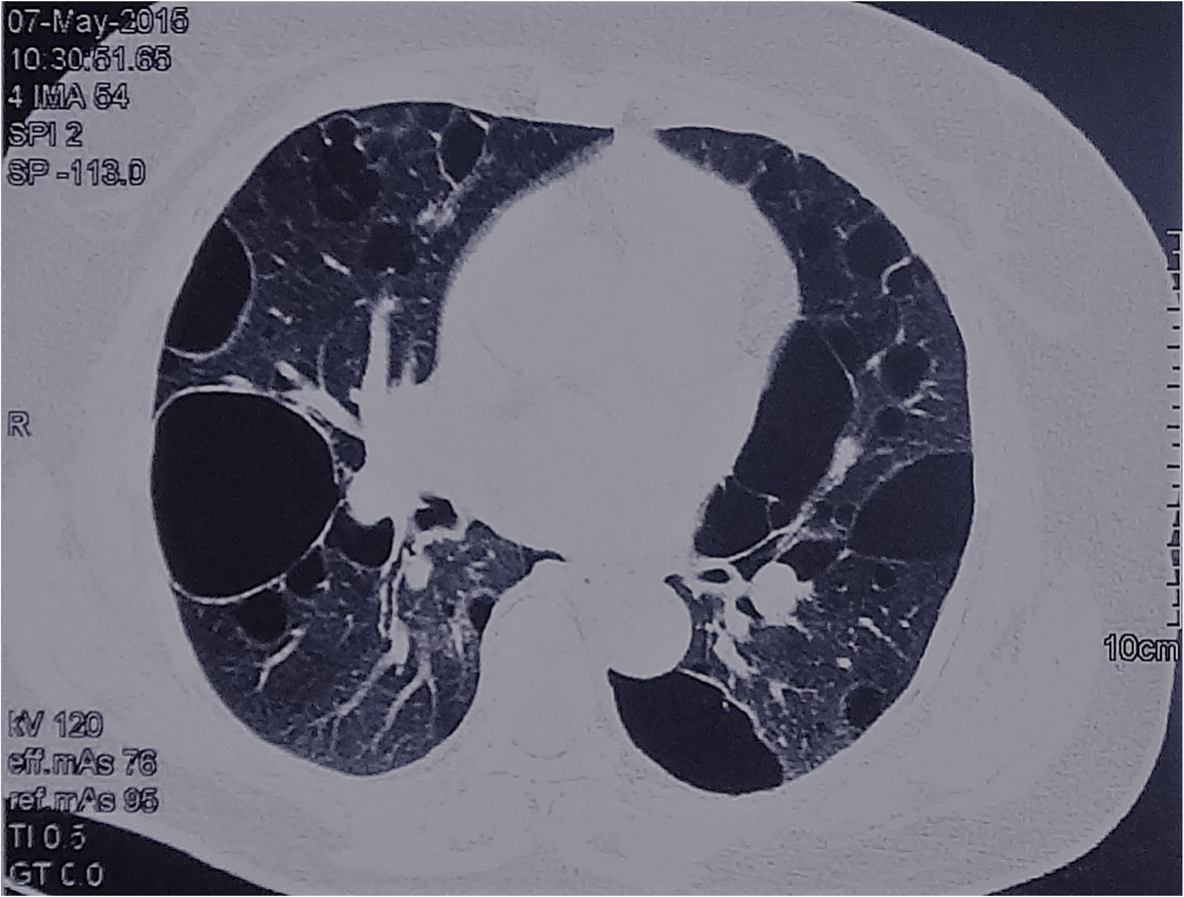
Chest CT showing multiple thin-walled cysts in Patient B24
